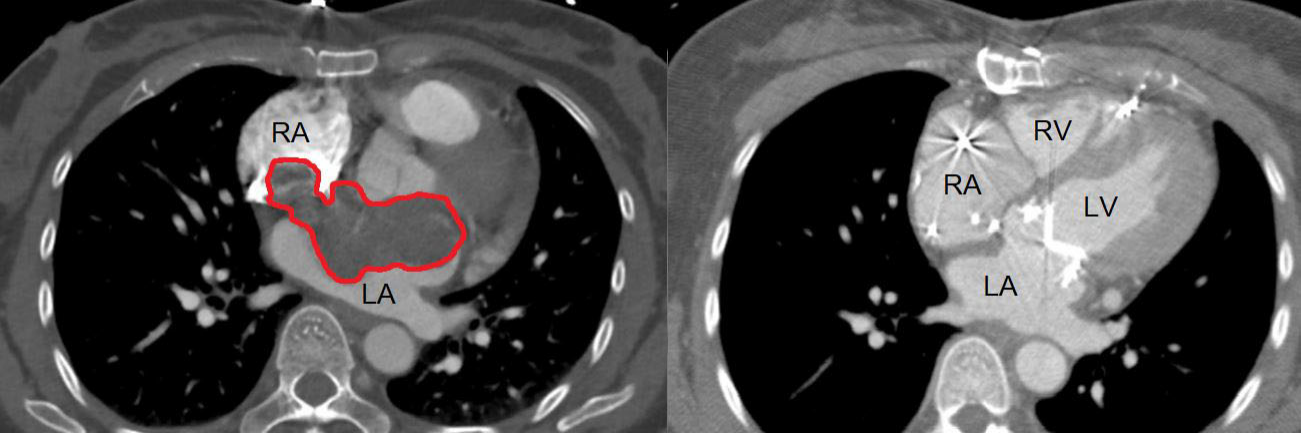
Preoperative (left) and postoperative (right) contrast-enhanced chest CT images on demonstrating a large filling defect (red) corresponding to the tumor mass involving the bilateral atria preoperative imaging, with resolution of the defect following surgical resection on postoperative imaging. LA, left atrium; RA, right atrium; LV, left ventricle; RV, right ventricle.
